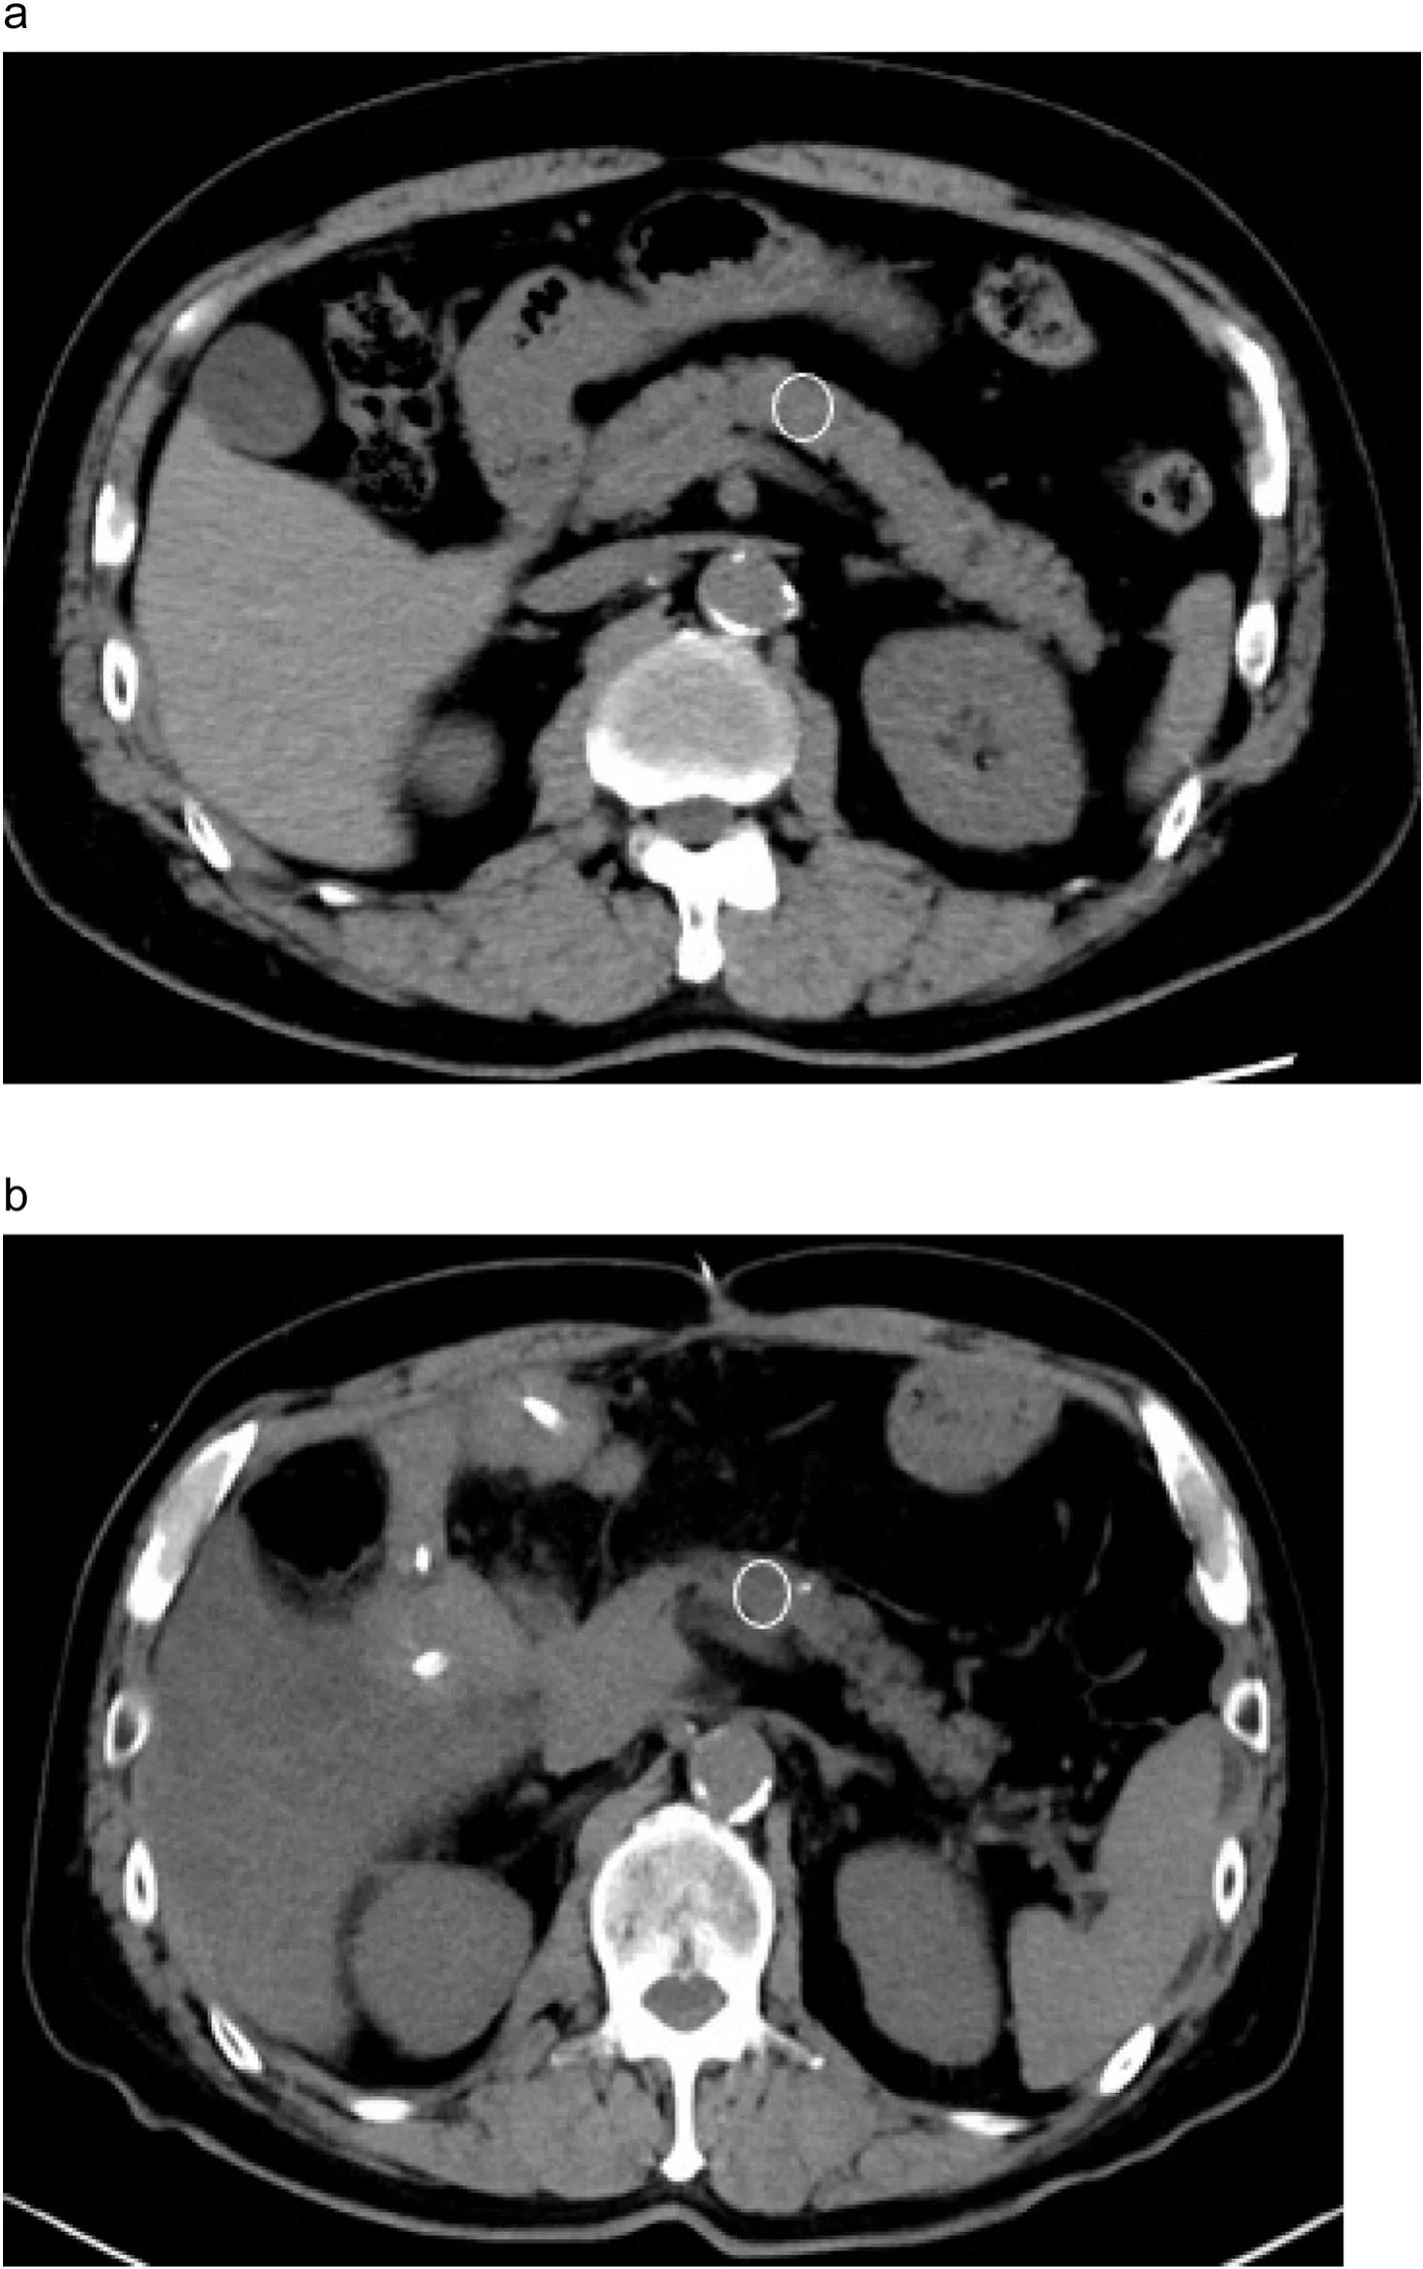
a Before initial PD, the CT attenuation value of the pancreas body was measured. Each region was a circular area 1.5 cm in diameter. b After initial PD, the CT attenuation values of the remnant pancreas was measured. Each region was a circular area 1.5 cm in diameter.3.The stent tube was passed into the jejunal lumen and externalized through the jejunal loop (Fig. 1d). The jejunal side of the tube was held in place with a purse-string suture. The stent tube was ligated with two stay sutures to approximate the pancreatic ductal cut end and the tiny hole in the jejunum (Fig. 1e).4.Finally, a suture was added between the anterior parenchyma of the pancreas and the seromuscular layer of the jejunum (Fig. 1f).
